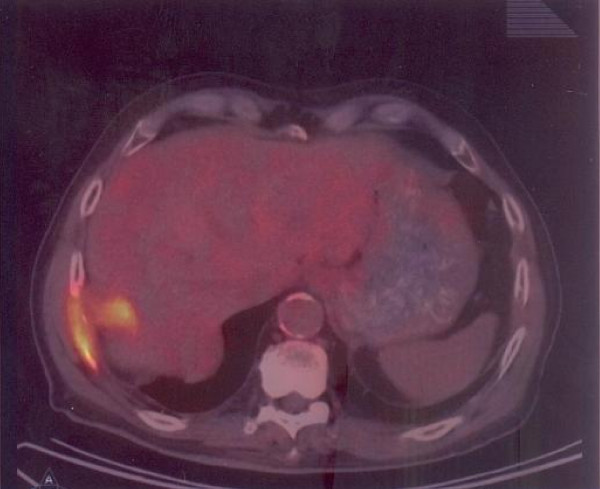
PET-CT. Isolated recurrence in chest wall of a previously resected cholangiocarcinoma: intense glucose uptake.
